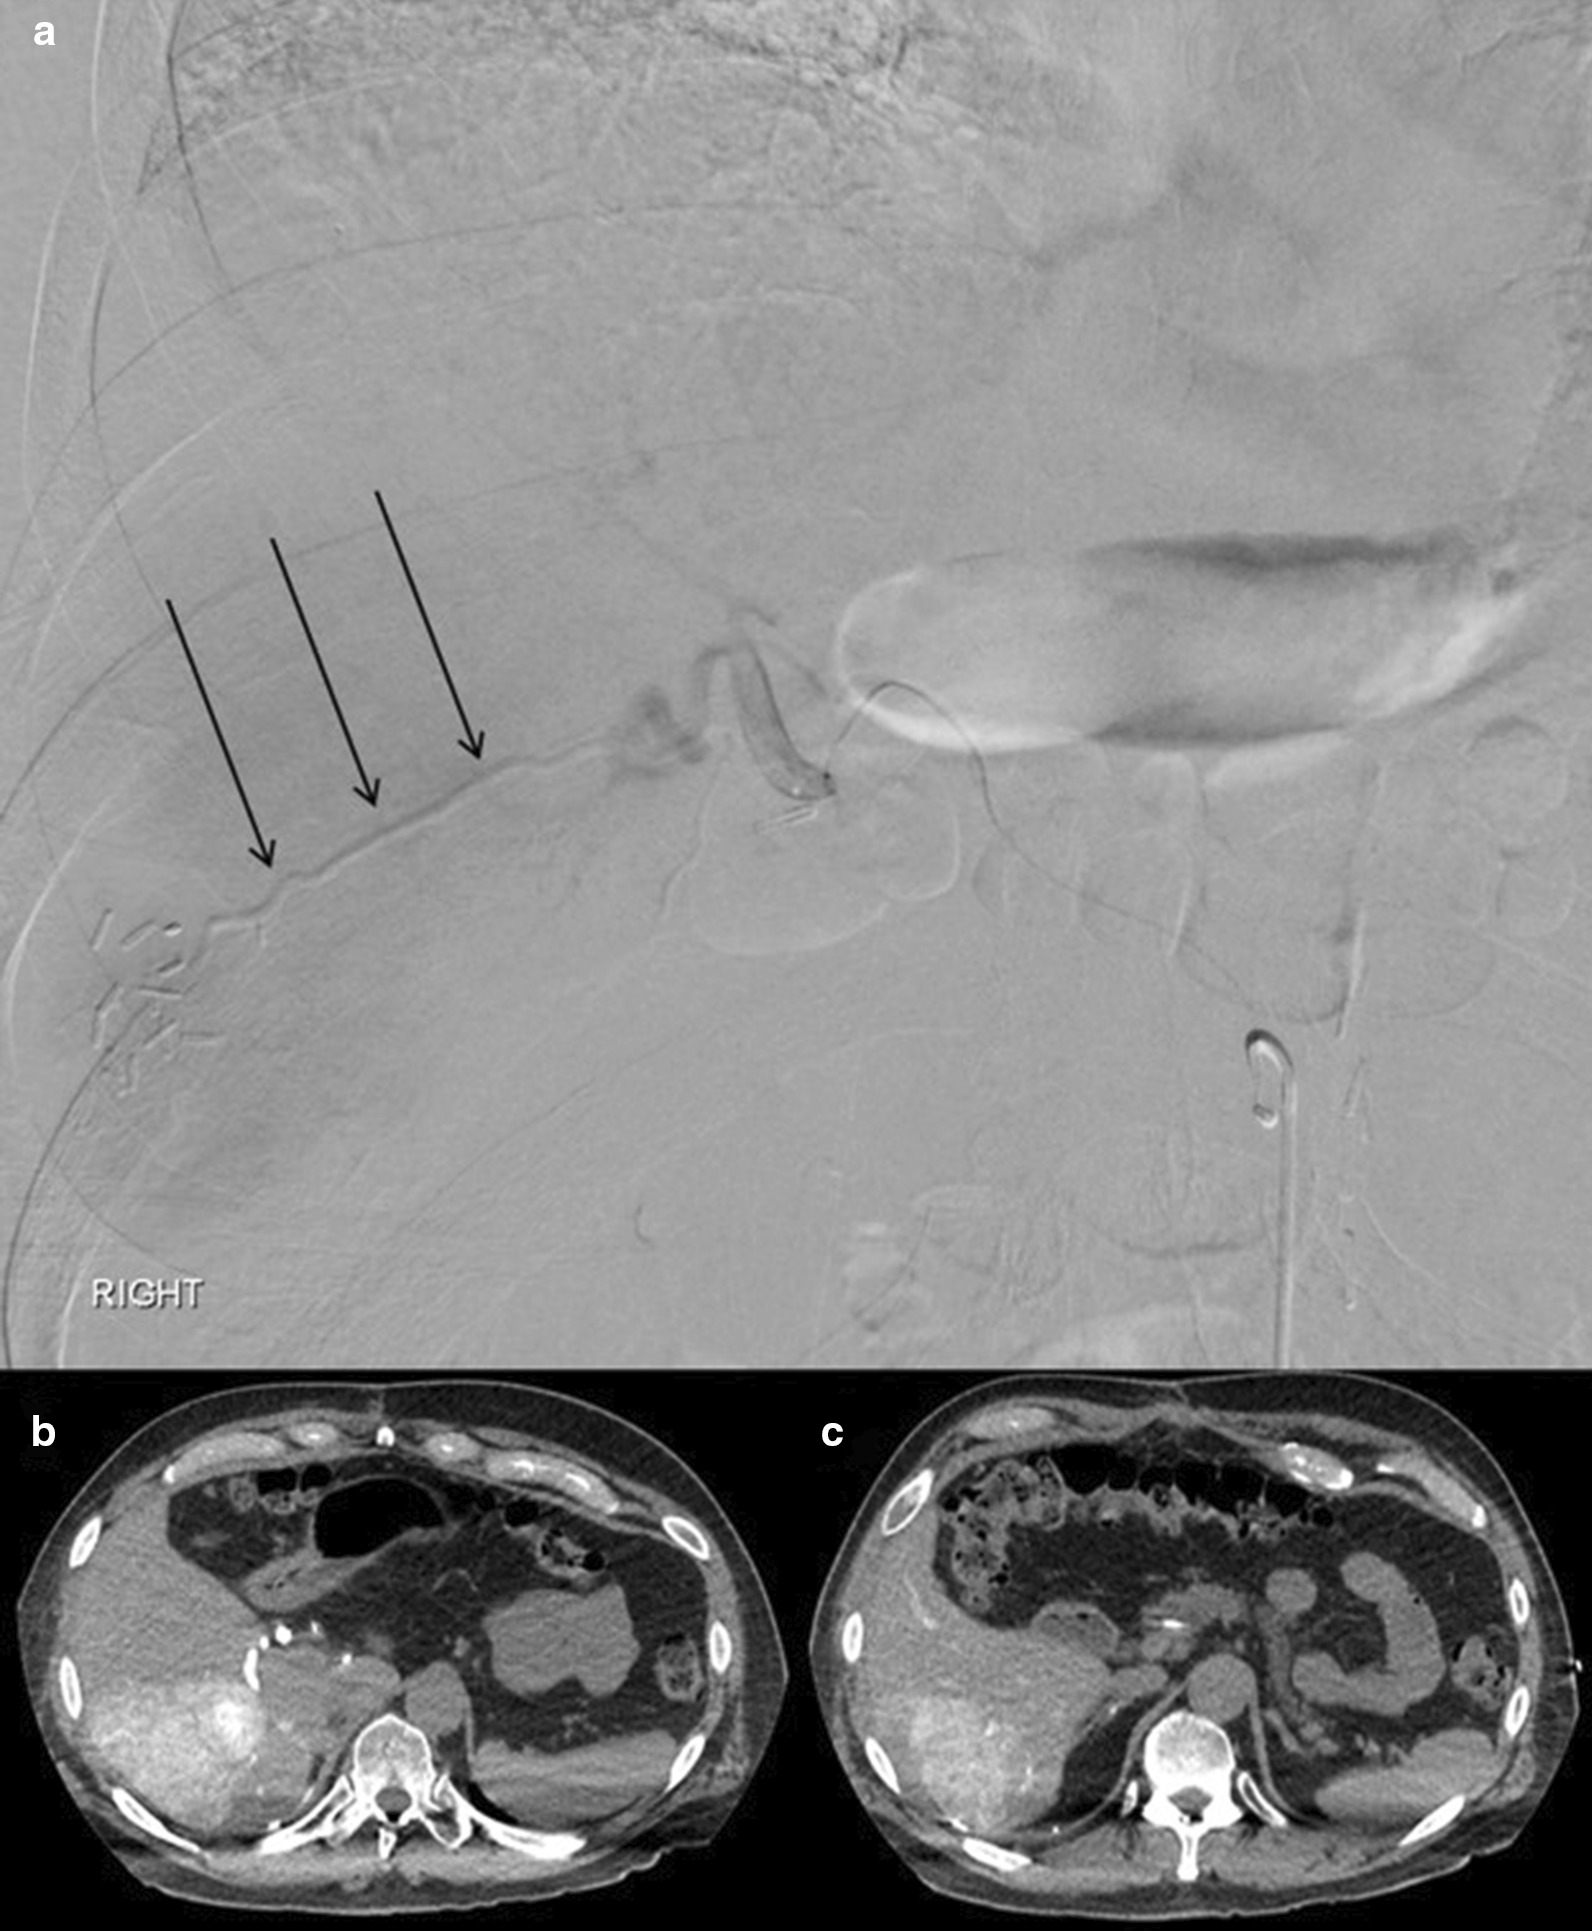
Pre-SIRT redistribution of flow. a Right hepatic artery arteriogram demonstrates the embolized inferior branch of the right phrenic artery, compatible with embolic material (arrows). b, c Intraprocedural selective 3D arterial-phase contrast-enhanced CT of the right hepatic artery demonstrates enhancement of the right hepatic lesions with perfusion of the tumors from the right hepatic artery instead of the right inferior phrenic artery branch that was bland embolized
